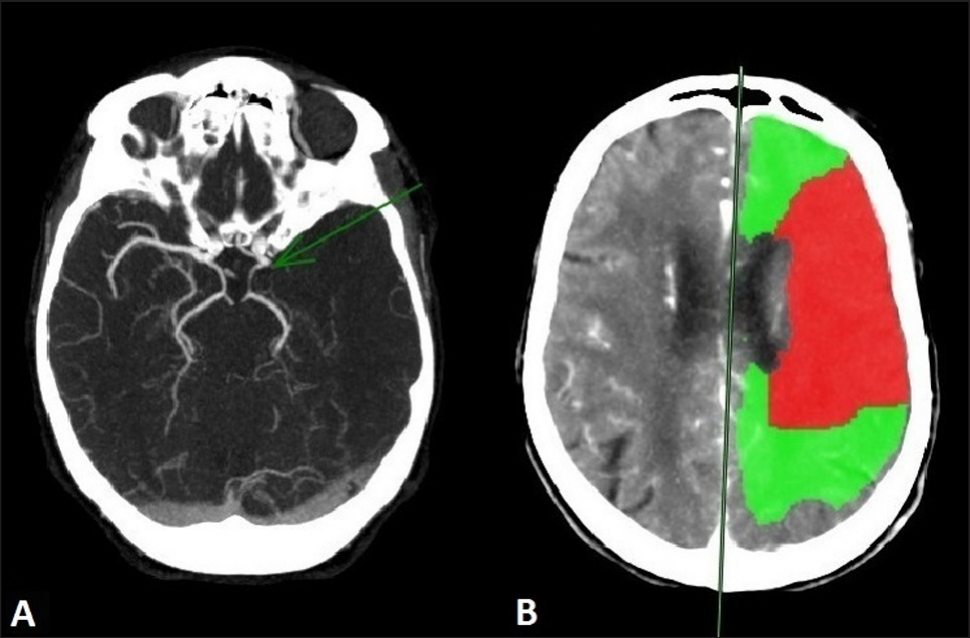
(A) CTA image showing proximal occlusion of the left MCA (green arrow) and (B) CTP image showing a mismatch between the infarcted core in red and the peripheral hypoperfusion in green.
